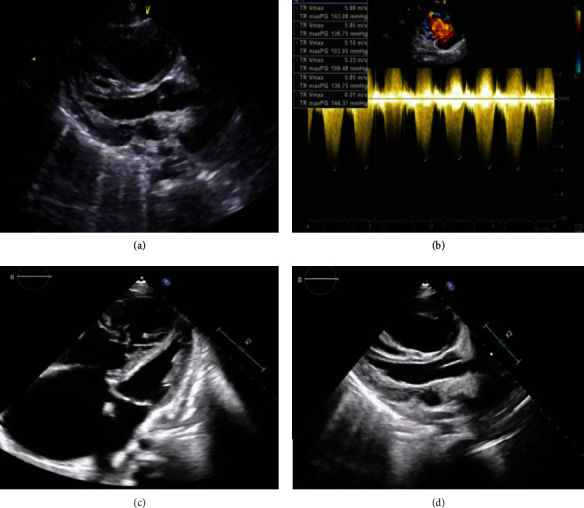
Postpericardiocentesis follow-up transthoracic (a, b) and transesophageal (c, d) echocardiograms showing (1) normal left ventricular dimensions and systolic function, (2) dilated right-sided chambers with reduced RV function, (3) severe functional TR, and (4) no residual pericardial effusion.
